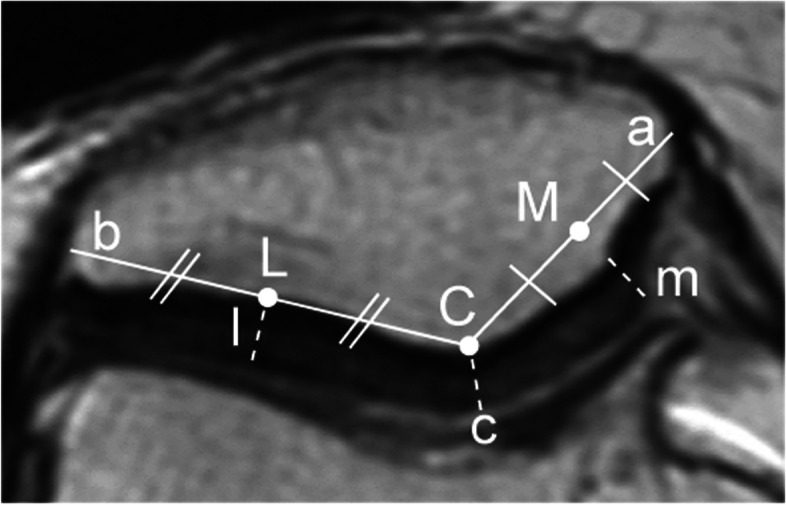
Method of measuring patellar cartilage thickness using an axial single MRI image [a] is a line from the medial edge of the patella to the central edge of the patella; [b] is a line from the lateral edge of the patella to the central edge of the patella; [C] is the central edge of the patella; [M] is the midpoint in line [a]; [L] is the midpoint in line [b]. Dotted lines [c], [m], and [l] represent the cartilage thickness in the central, medial, and lateral facets, respectively. MRI, magnetic resonance imaging
